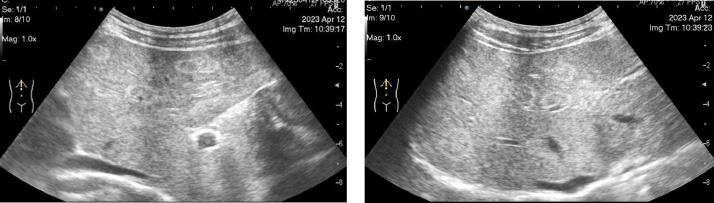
Liver ultrasound reveals multiple centimetric nodules scattered throughout the liver parenchyma.
